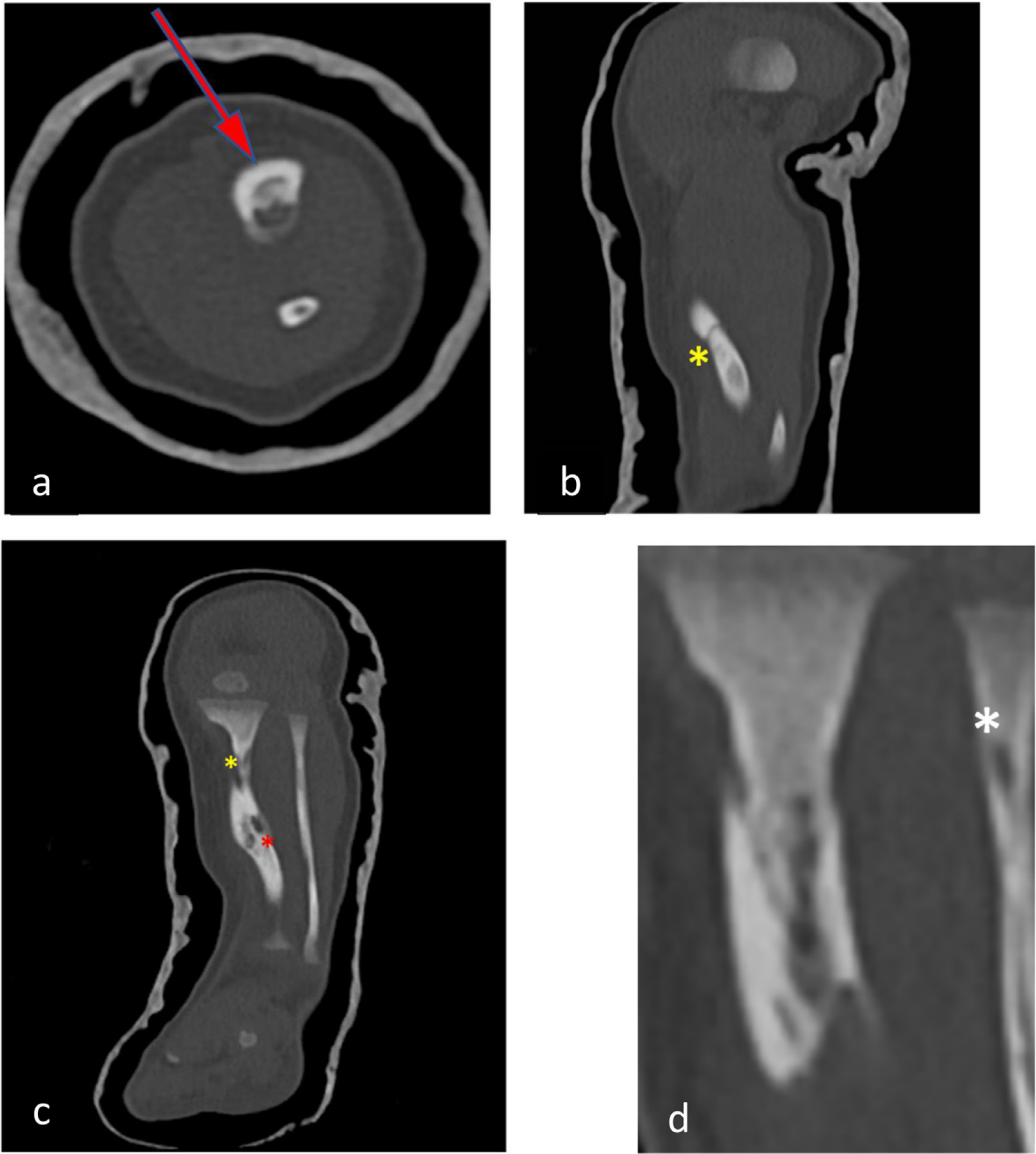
CT scan of the left lower limb shows (A) thickening (arrow) and (B and C) discontinuities of the bone cortex in the mid-diaphyseal regions of the tibia (yellow asterisks). (C) Multiple irregular hypodense areas are present along the tibia, extending to the bone cortex (red asterisk). (D) The fibula exhibits a small area of hypodensity in the proximal diaphyseal region, altering the cortical profile (white asterisk).
